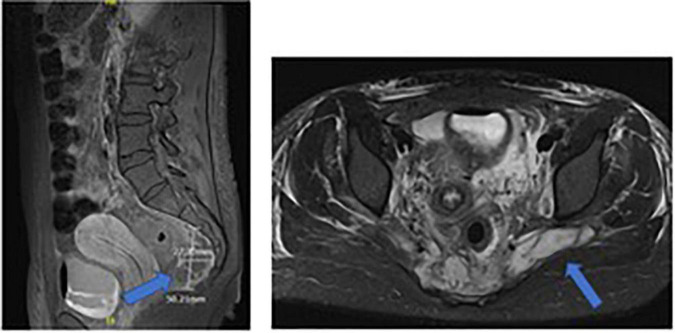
Sacral MRI of case 3: sacral osteomyelitis and multiple adjacent abscesses.
